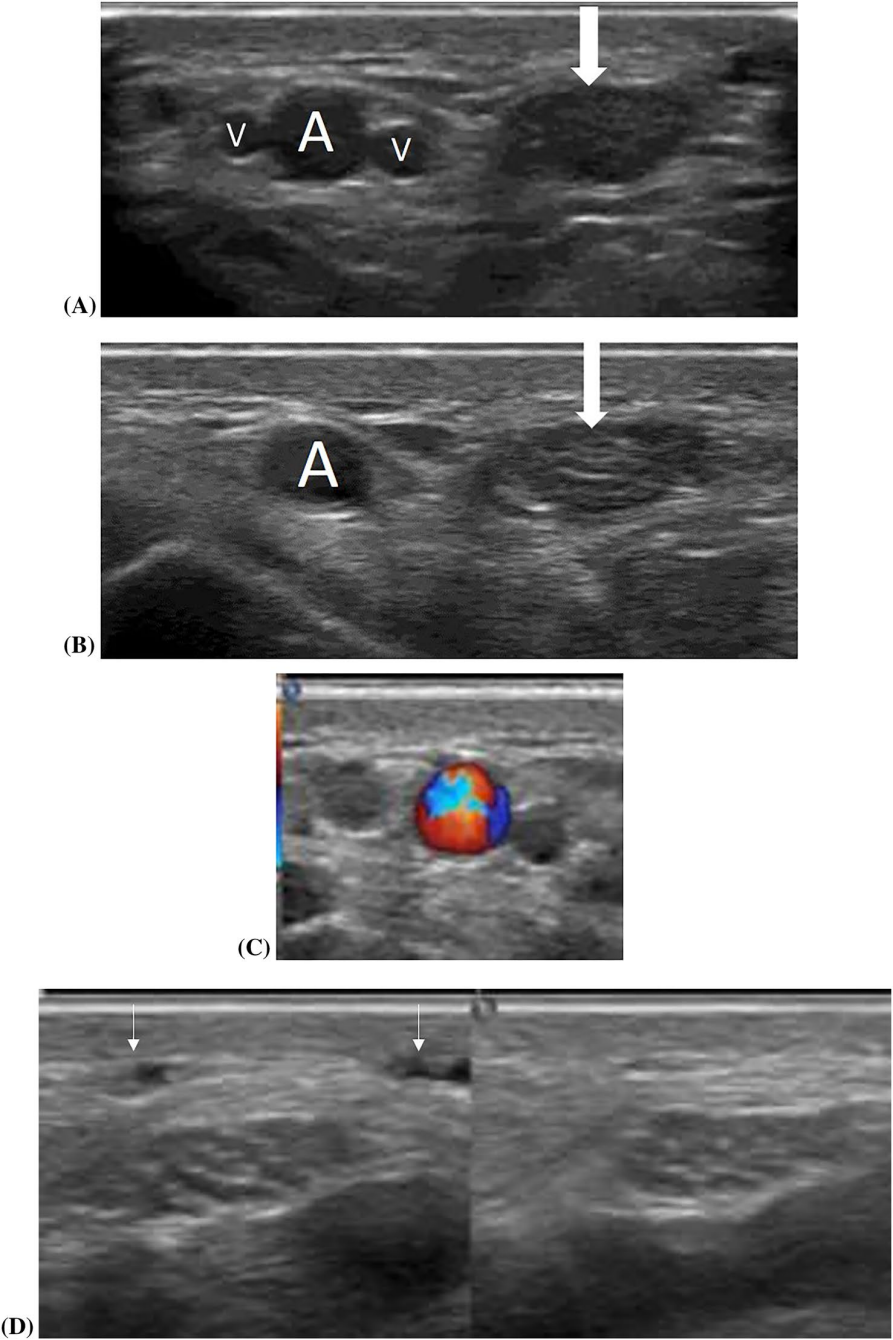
Displays compression and Doppler interrogation abilities to differentiate between arteries, veins, tendons, and nerves. Light probe touch in A and gentle compression in B, compression reveals total obliteration of the venae comitantes (v), subtle effacement of the radial artery (A), and non-deformity of the median nerve (thick arrow) in both A and B. Color saturation on C helps distinguish arteries (asterisk) from nerves and tendons. D Subcutaneous veins respond to compression, while the median nerve does not
